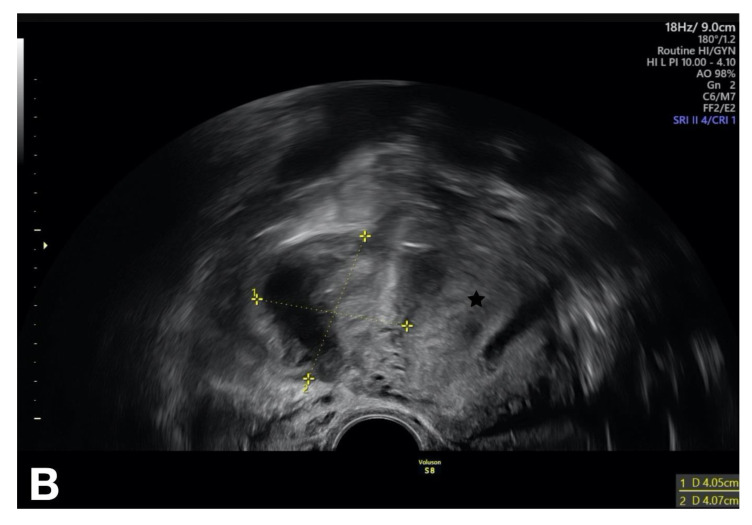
Vaginal ultrasound investigation showing the tubo-ovarian abscess in the right-sided adnexa. The uterus is marked with a star [5].
